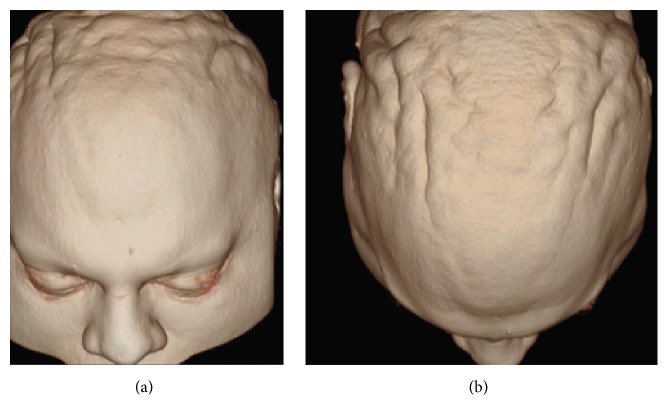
((a) and (b)) Presence of* cutis verticis gyrata* proven in this MRI image.
